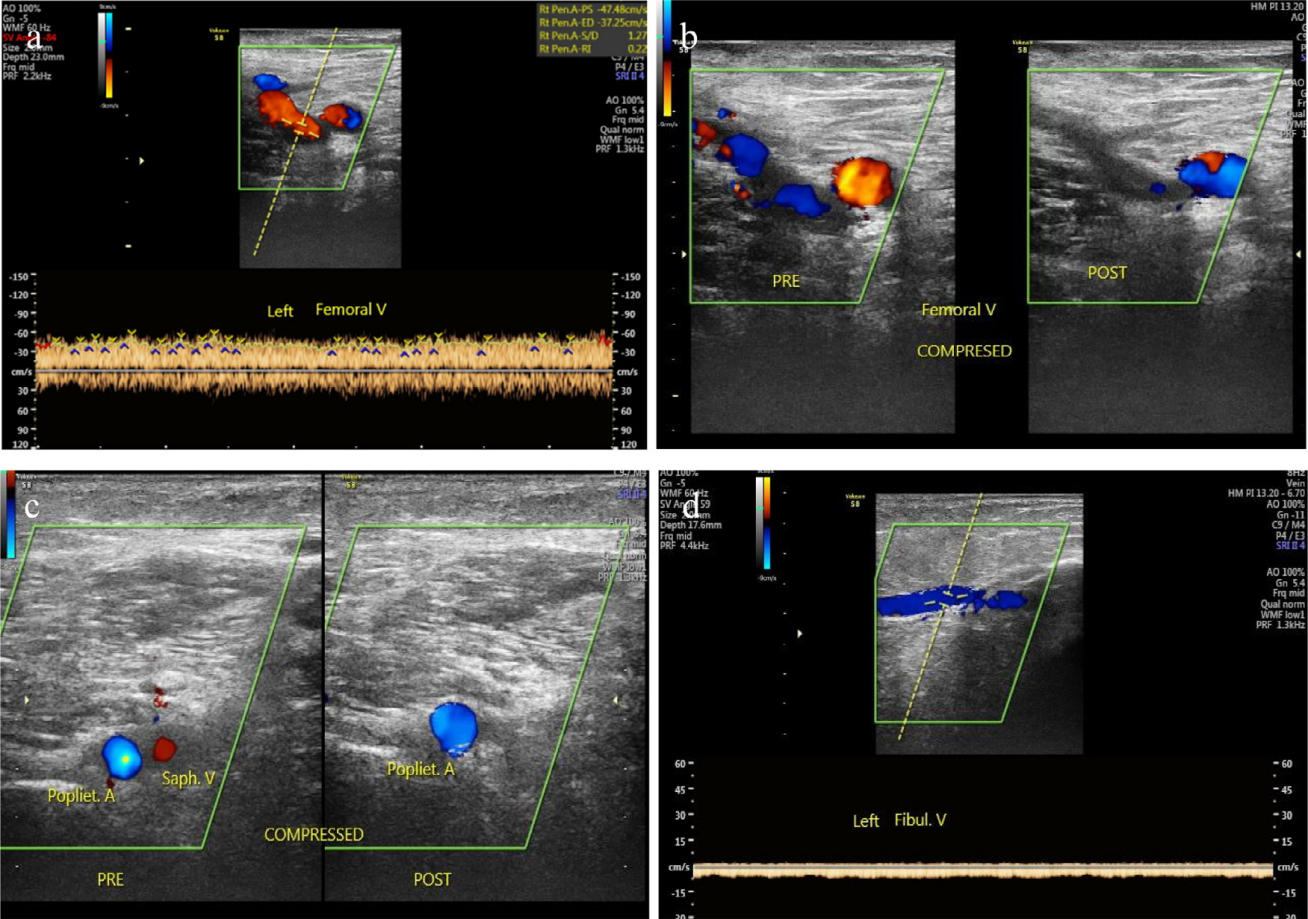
Doppler ultrasound on the left femoral vein (A), compressible left femoral vein (B), left popliteal vein (C), and left fibular vein (D) without any thrombus or stenosis 3 months after the treatment.
